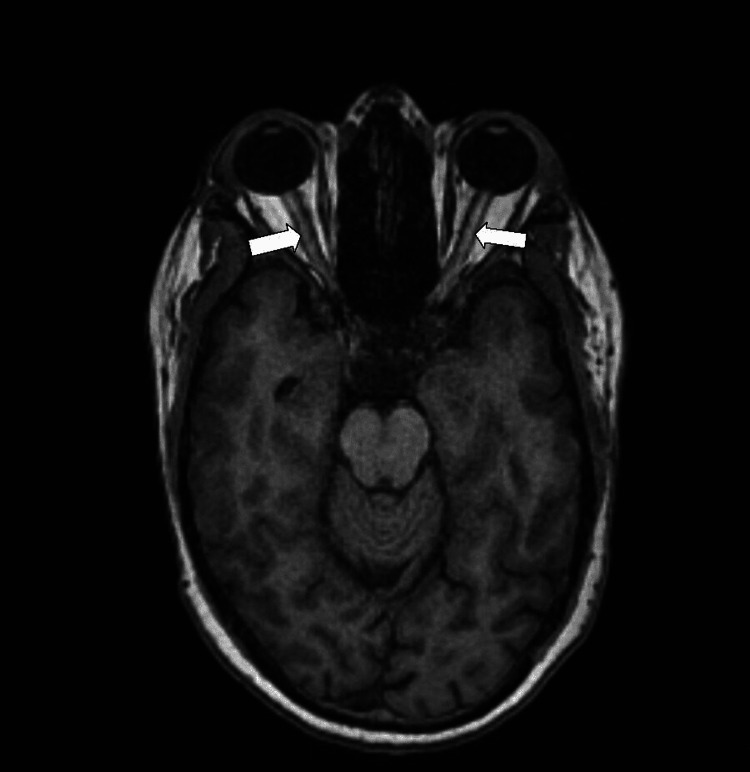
Axial T1-weighted image showing bilateral optic nerve heads flattened and distended bilateral optic nerve sheaths shown by white arrows.
